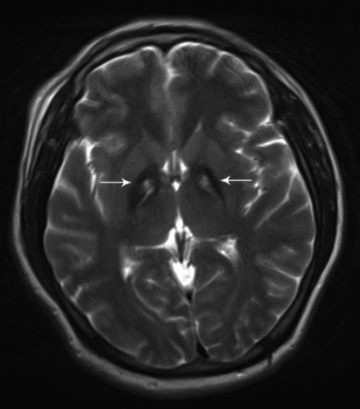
T2-weighted brain MRI of the 39-year-old patient showed bilateral symmetrical hypointensity in the globus pallidus with central hyperintensity, giving an eye-of-the-tiger sign (arrow).
